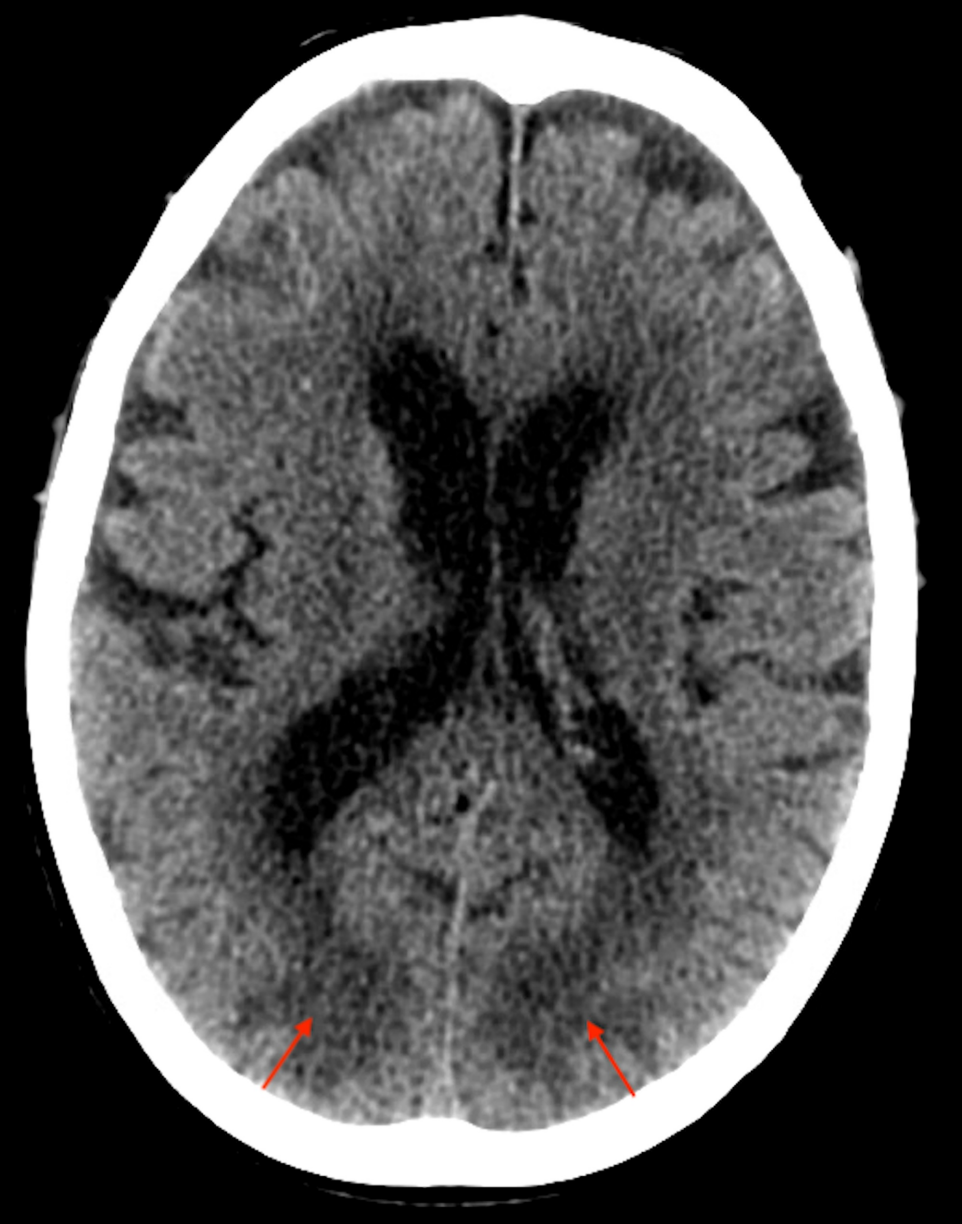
CT of the head reveals low attenuation changes in occipital lobes
